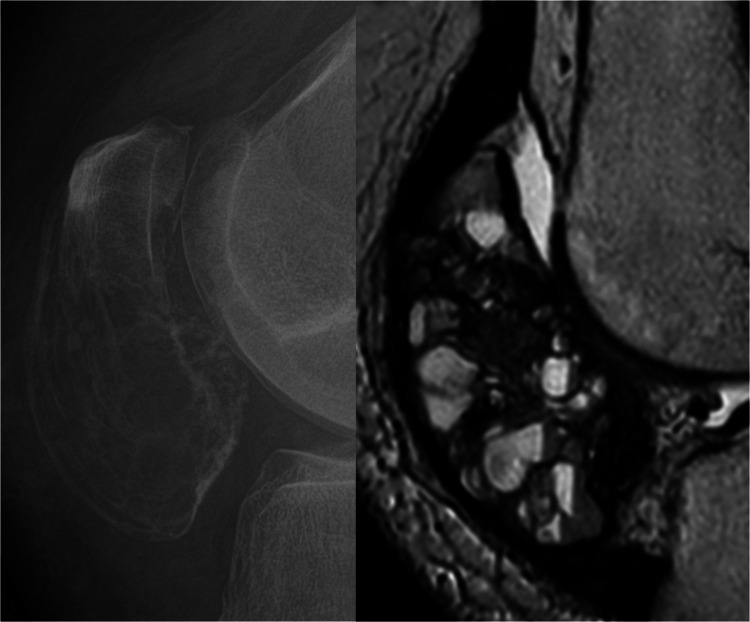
Fifty-five-year-old female with patellar giant cell tumour. Lateral knee radiograph (left) demonstrates a markedly expansile lytic patellar lesion with prominent associated cortical attenuation and effacement of the infrapatellar fat pad. Sagittal T2-weighted image (right) shows ABC-like changes with multiple fluid–fluid levels
